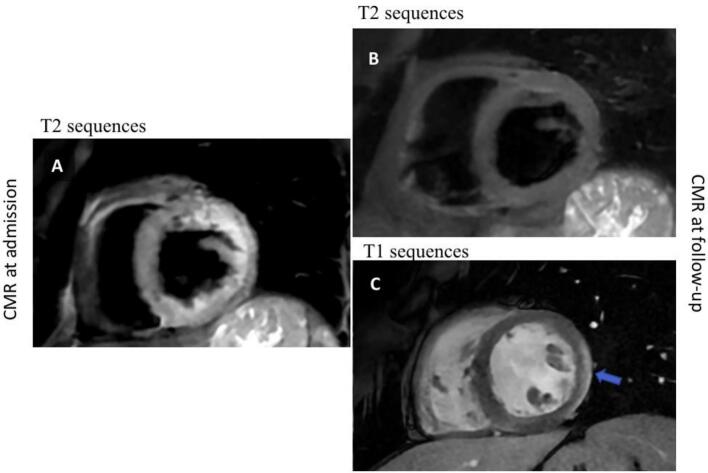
Cardiac magnetic resonance imaging studies. A. CMR of the patient at admission, showing an extensive oedema at T2 sequences at the mid and apical segments of the lateral and inferior walls. The initial LVEF was 25%. B-C. Follow-up CMR showing the absence of oedema in the T2 sequences (B) and a multifocal subepicardial LGE within the lateral wall, as indicated by the arrow (C).
